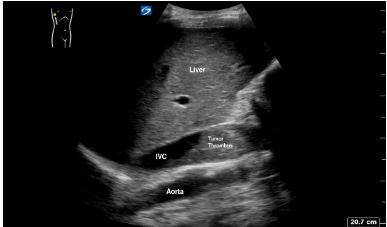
Point-of-care ultrasound of the abdomen demonstrating a hyperechoic, heterogeneous structure in the inferior vena cava.
